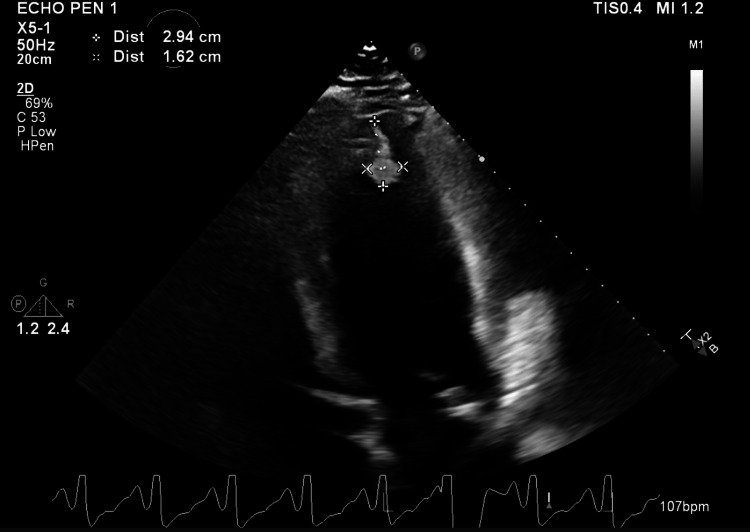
Echocardiography showing presence of apical mural thrombus
